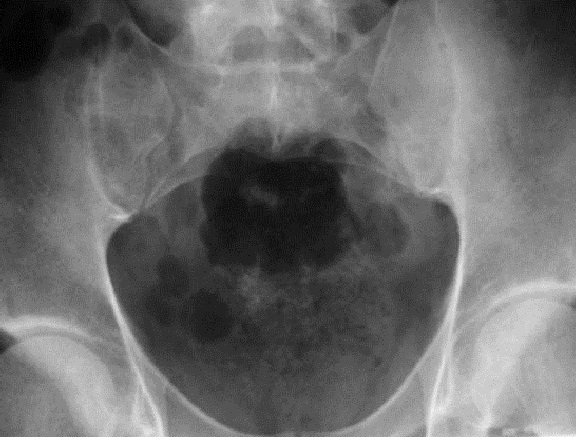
Radiographie standard du bassin de face; Aspect flou condensé des berges de la sacroiliaque gauche; Aspect normal de la sacroiliaque droite
